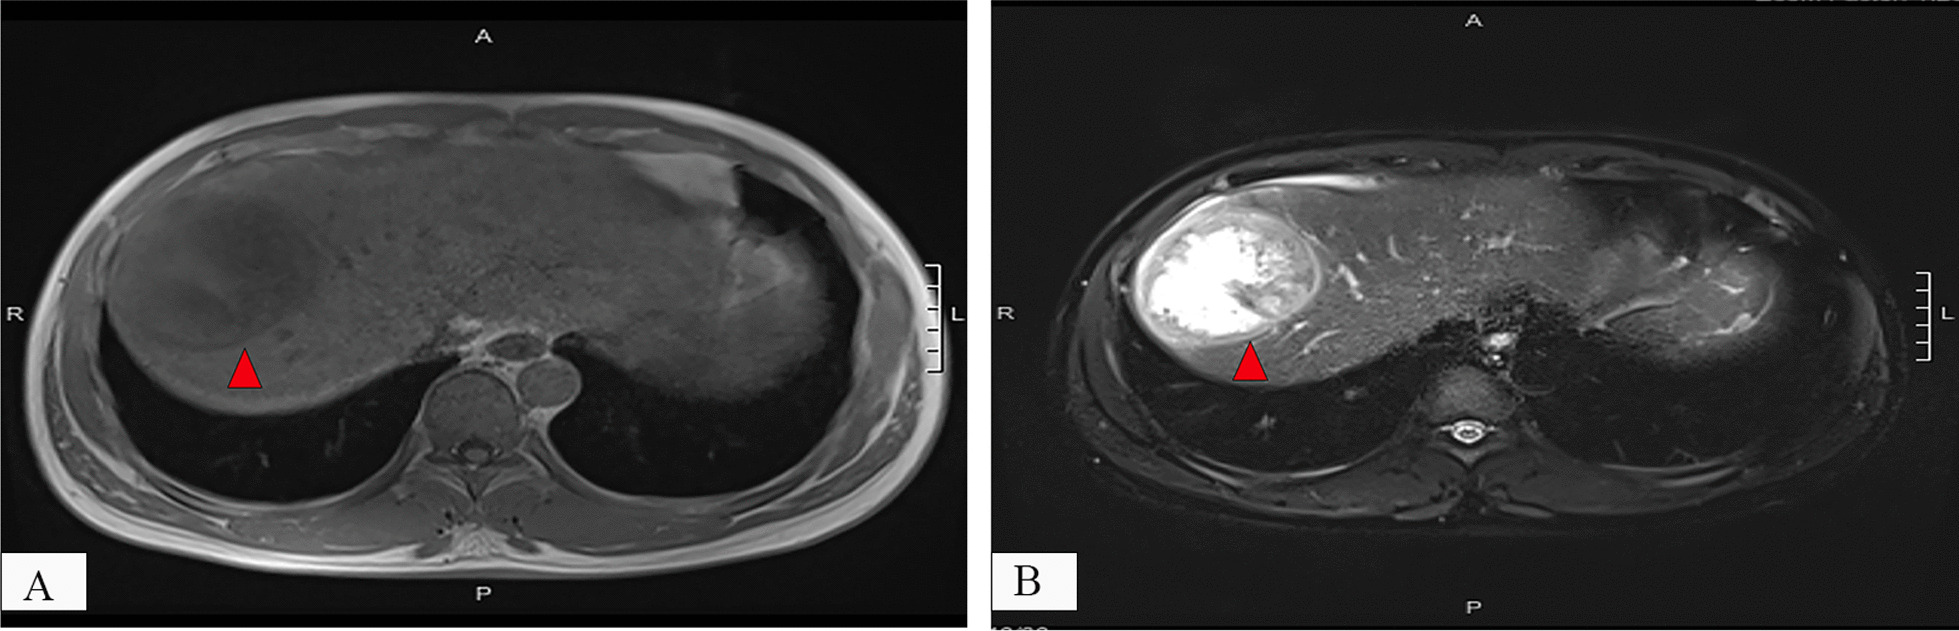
Abdominal MRI findings in HCC with OGCs. T1-weighted image showing a round-shaped hyperintense lesion (red arrow) with heterogeneous internal signal intensity (A). After contrast enhancement, it demonstrates a ring-enhancing pattern (red arrow) (B)
